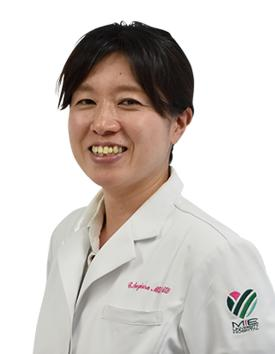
Dr Sugiura received her medical degree and completed her specialization in cardiovascular medicine at the Mie University Graduate School of Medicine in Japan. She is currently working as an echocardiologist at Mie University. Her areas of interest include echocardiography and structural heart interventions.
